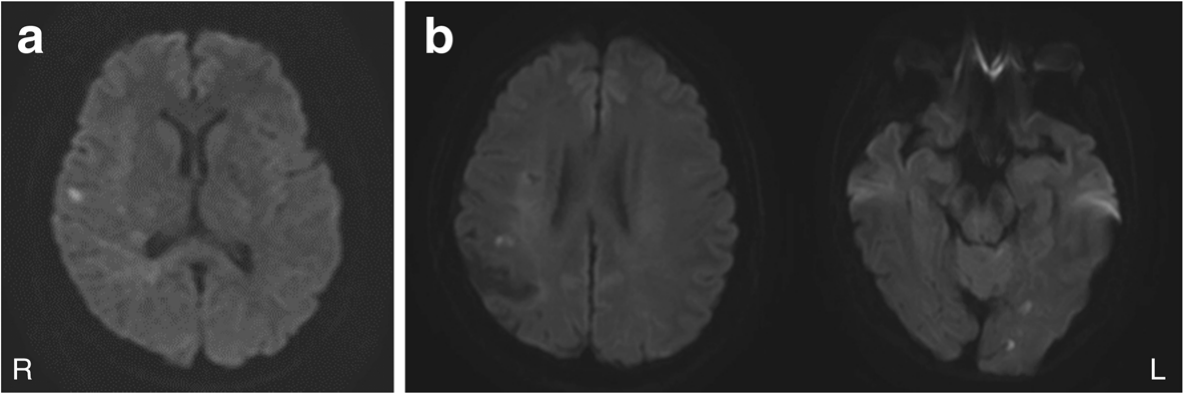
Diffusion-weighted MRI revealed CIs in the right cerebrum (a). Multiple cortical and subcortical CIs in the left occipital lobe and right parietal lobe (b)
